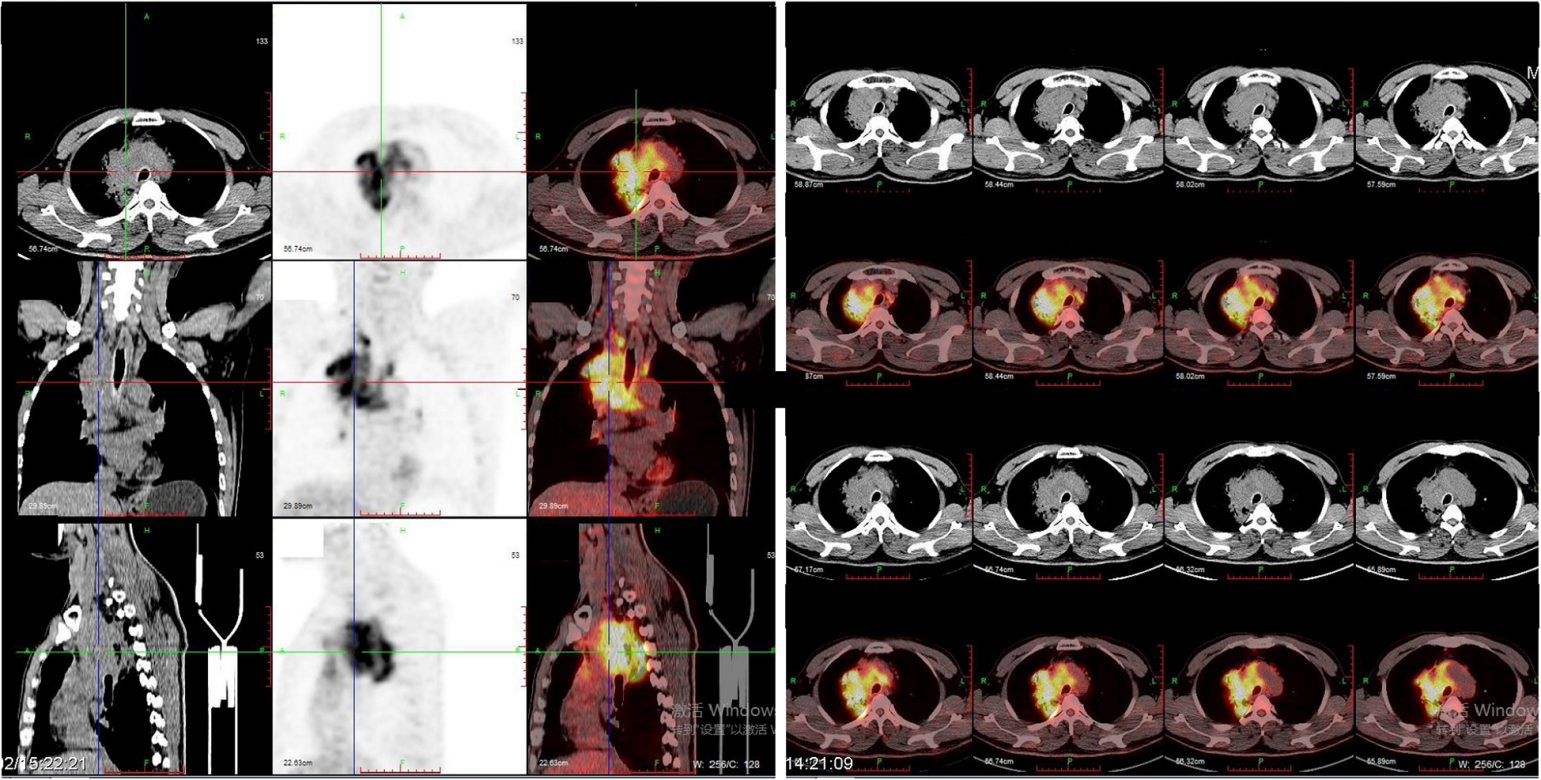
PET\CT images showed a mass (about 7.2 x 6.7 x 6.1 cm) in the superior lobe of right lung with the SUVmax was about 13.4 and lymph node metastasis in hilum of right lung, mediastinum, and right subclavian fossa.
